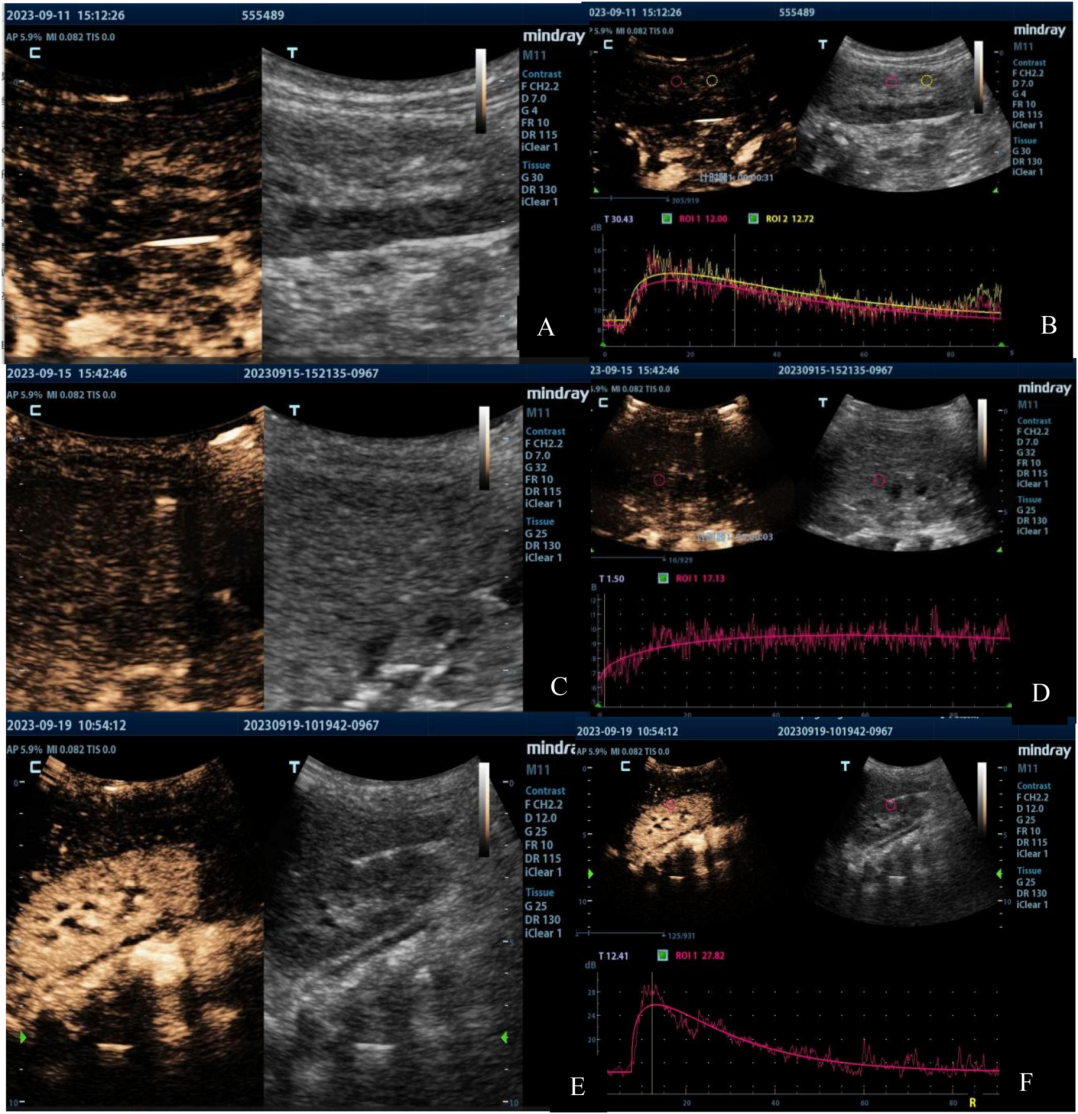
Contrast-enhanced ultrasound images of the left kidney in the first patient taken on Day 4 (A,B), Day 8 (C,D), and Day 12 (E,F). The images demonstrate a significant decrease and abnormal prolongation of perfusion time, followed by marked improvement by Day 12.
